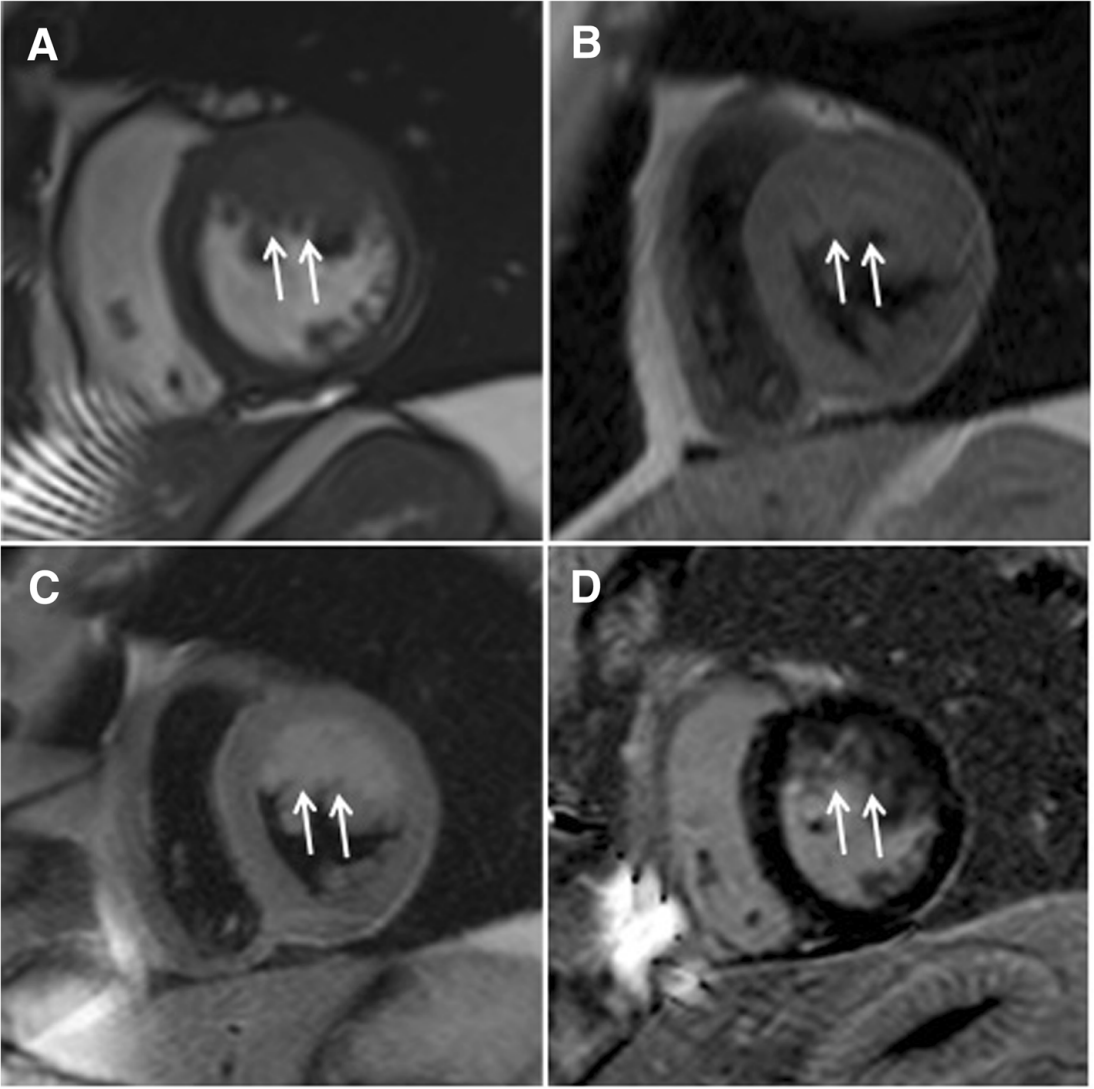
CMR examination before surgery. The cine image (a) demonstrates tumor (arrows) in the LV adherent to the anterior and anterolateral walls. Mass (arrows) appears isointense in T1-weighted (b), hyperintense in T2-weighted (c) and heterogeneously enhanced in late gadolinium enhancement (d) images
